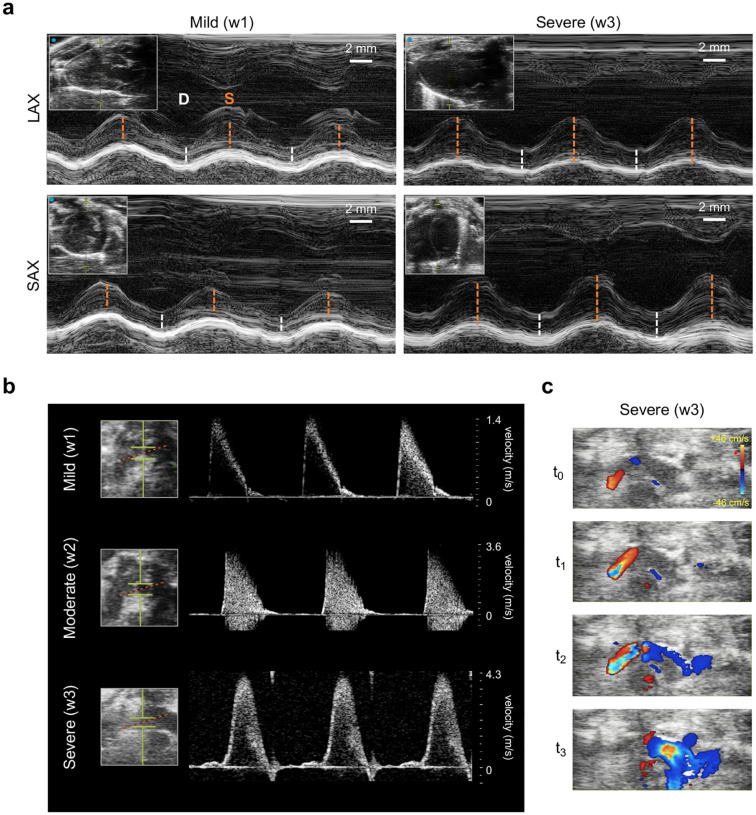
Examples of ultrasound structural and functional characterization of aortic banding model. a, Representative M-mode images of the LV in long-axis (LAX) and short-axis (SAX) at mild (w1) and severe (w3) aortic banding. Dashed lines indicate wall thickness during systole (S) and diastole (D). b, Representative pulsewave (PW) imaging of blood flow through the soft robotic actuator for mild (w1), moderate (w2), and severe (w3) aortic banding. c, Representative color Doppler imaging at four progressive time points (t0-t3) for severe aortic banding (w3). LAX = long-axis view; SAX = short-axis view; D = diastole; S = systole.
